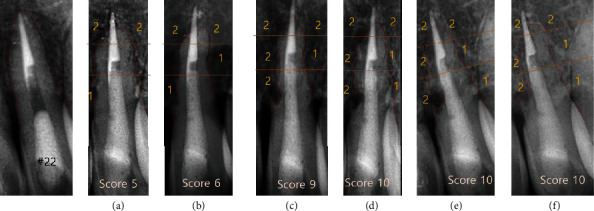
Radiographic index of tooth #22 ((a) 1 year; (b) 2 years; (c) 3 years; (d) 4 years; (e) 4 years: 10 m; (f) 9 years) (Andersson et al.) [14].
